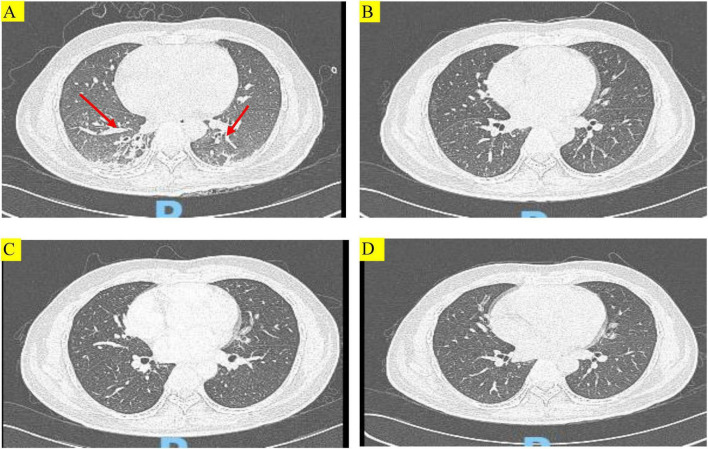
Chest computed tomography (CT) images of the patient during treatment. (A) Chest CT showing multiple patchy and striped shadows (red arrows) before treatment with TMP-SMX. (B–D) Chest CT scans conducted at 14 days after treatment with TMP-SMX, 29 days after discharge, and 57 days after discharge, respectively. Multiple patchy and striped shadows were not visible in both lungs after treatment.
